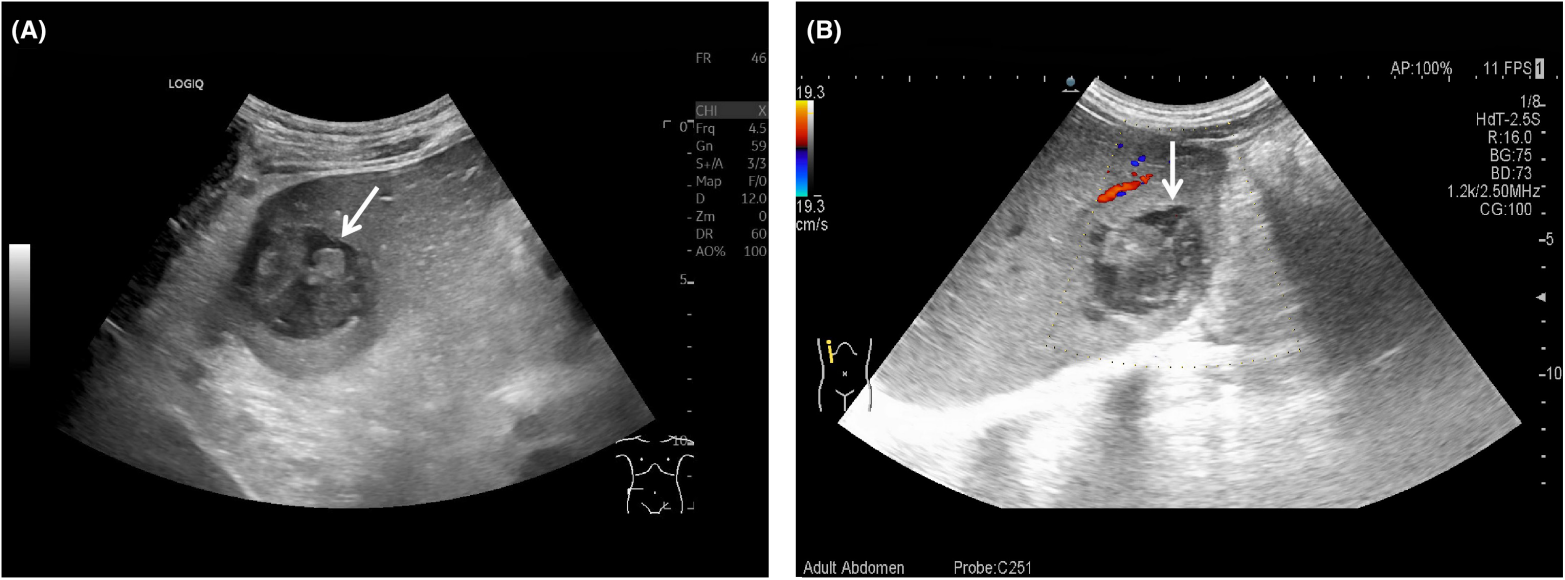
Routine abdominal ultrasound of sarcomatoid hepatocellular carcinoma. (A) Grayscale ultrasound showed a cystic‐solid mass (arrow) located in the right hepatic lobe that was irregular, ill‐defined, inhomogeneous, and approximately 56 × 53 × 44 mm in size. (B) Color Doppler flow imaging showed no blood flow signal inside the mass (arrow).
